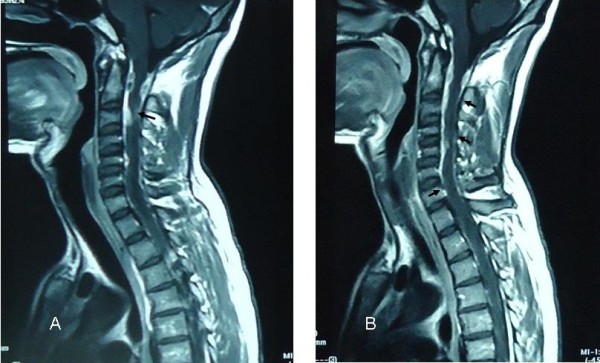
T1-weighted magnetic resonance imaging revealing an intra-medullary tuberculoma. Sagittal sections; multiple hyper-intense lesions are visible at C1 (A) (arrow) and (B) (top arrow), C2 and C6 levels (B) (bottom two arrows).
